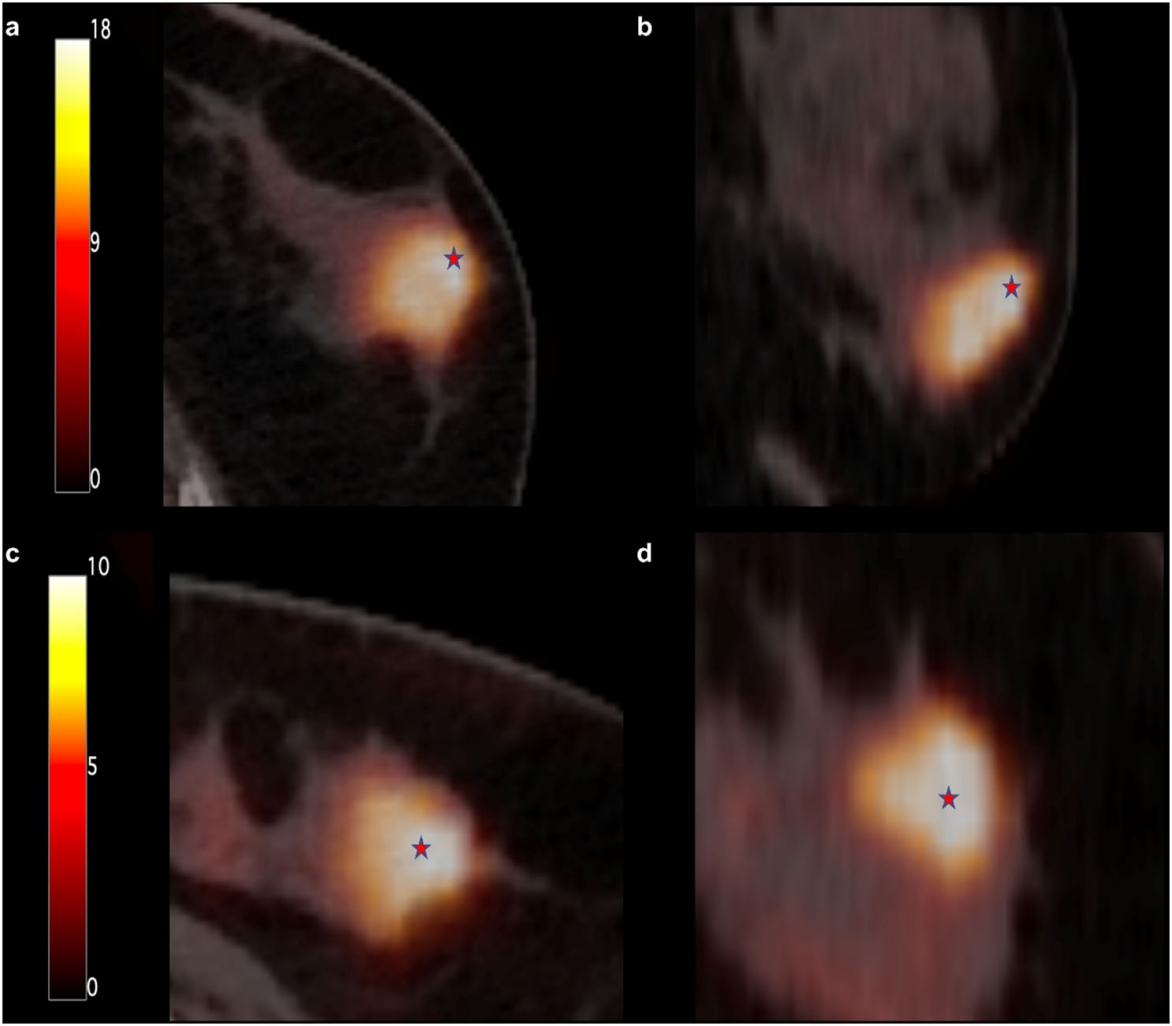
Transaxial (A) and coronal (B) FDG PET/CT images of a 52-year-old woman who was a non-responder to NAC with residual tumor cellularity of 50%. Her values of SUVmax, MTV, NHOCmax, and NHOCpeak were 21.63, 9.06, 0.78, and 0.75, respectively. Transaxial (C) and coronal (D) FDG PET/CT images of a 54-year-old woman who showed pCR to NAC. Her values of SUVmax, MTV, NHOCmax, and NHOCpeak were 10.83, 7.67, 0.27, and 0.23, respectively. Position of hot spot of FDG uptake is indicated by a red star
